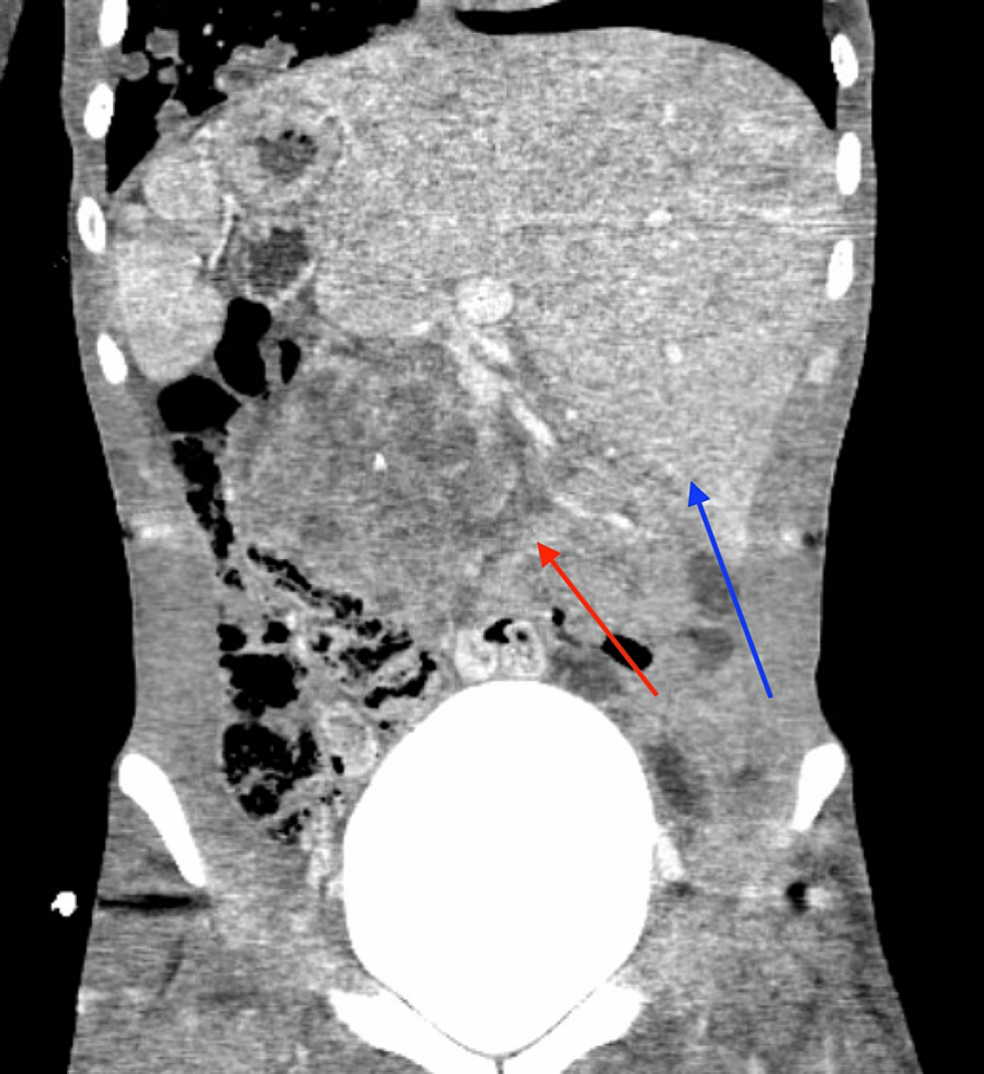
Abdominal CT. Red arrow pointing at the 9-cm retroperitoneal mass Blue arrow pointing to the liver on the left side of the abdomen consistent with the patient's abdominal heterotaxy
